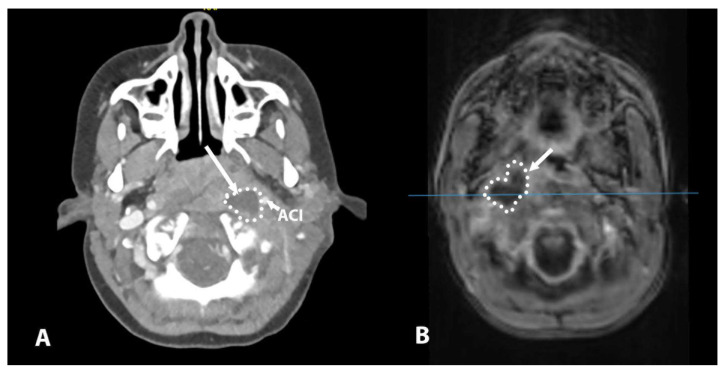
(A) CT scan showing a collection medial to the vascular structures (ACI) on the left side. (B) MR scan showing a collection medial to the vascular structures; note inferior image quality due to the child’s movement during the scanning procedure, despite sedation. In these cases, the route to the collection was achieved through the pharynx to avoid injury to the vessels. ACI—internal carotid artery.
